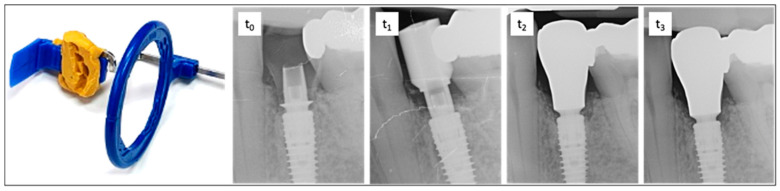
On the left is the radiographic film holder, which was customized with malleable silicone to ensure a reproducible position for all imaging sessions. On the right is an exemplary sequence of dental radiographs taken at time points t_0_, t_1,_ t_2_, and t_3_. t_0_—time of implantation. The subcrestal position of the implant and bonding base is clearly visible. Adaptation using the Bone Profiler System enabled the insertion of the provisional restoration by removing obstructive proximal bony overhangs and shaping the emergence profile. t_1_—six months after implant placement with a scan body. t_2_—one-year follow-up showing bone remodeling and the final restoration. t_3_—two-year follow-up demonstrating stable bone conditions around the implant and completed remodeling.
